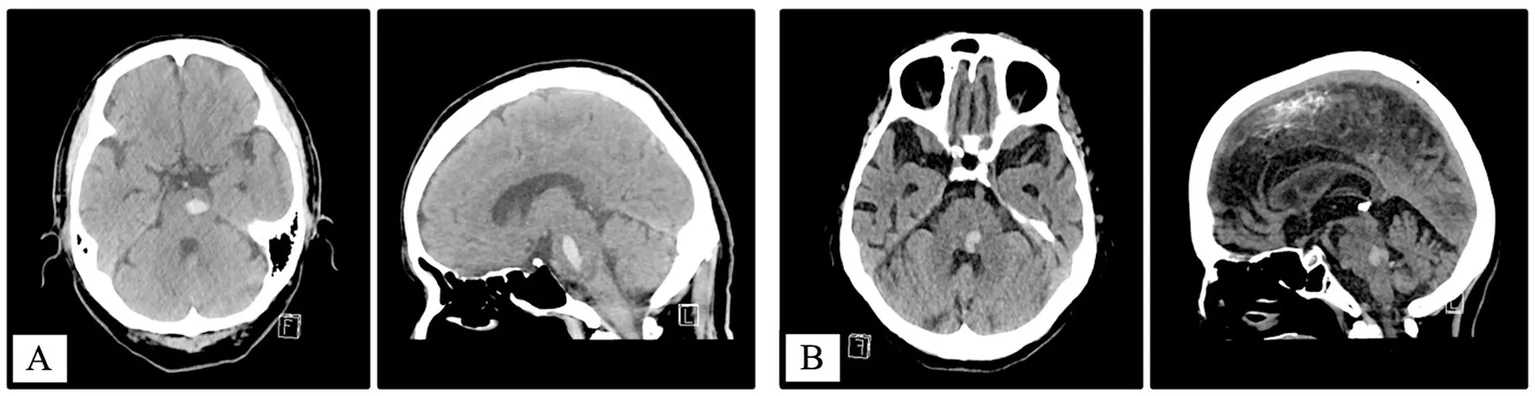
CT imaging of isolated infratentorial hemorrhage in two patients with more severe symptoms. (A) Axial and sagittal CT images of a 65-year-old male patient (NIHSS score of 35) presenting in a comatose state, showing a brainstem hemorrhage within the basal pons with a volume of 1 mL. (B) Axial and sagittal CT images of an 88-year-old female patient (NIHSS score of 8), demonstrating a brainstem hemorrhage located in the pontine tegmentum with a volume of 0.5 mL. Both cases highlight the small volume and severe clinical presentation of isolated infratentorial hemorrhage. NIHSS, National Institutes of Health Stroke Scale.
